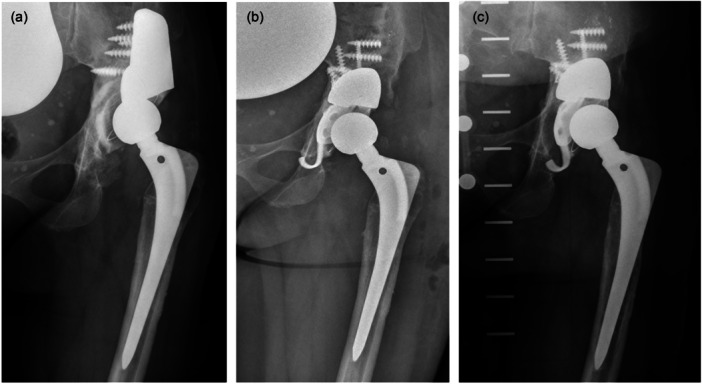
(a) Preoperative anteroposterior radiograph of the hip of a 50‐year‐old patient with severe acetabular bone loss due to aseptic loosening of the cemented cup. (b) Anteroposterior radiograph of the same patient postoperatively, with a modular TM augment in combination with a Ganz reinforcement ring and a cemented PE cup. (c) Anteroposterior radiograph 4.7 years postoperatively, with signs of loosening and dislocation of the reconstruction ring and changed cup position. There are no signs of loosening of the TM augment. TM, trabecular metal.
